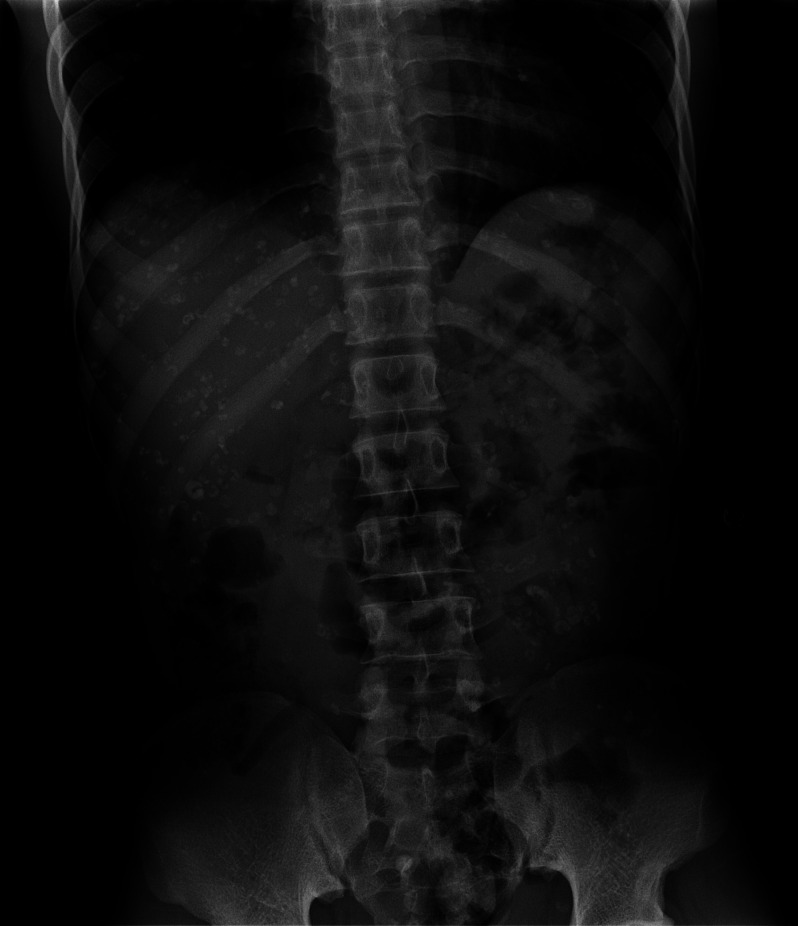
Abdominal X-ray of a young male patient showing a large number of horseshoe-shaped calcifications in the peritoneal cavity, indicative of pentastomiasis.
